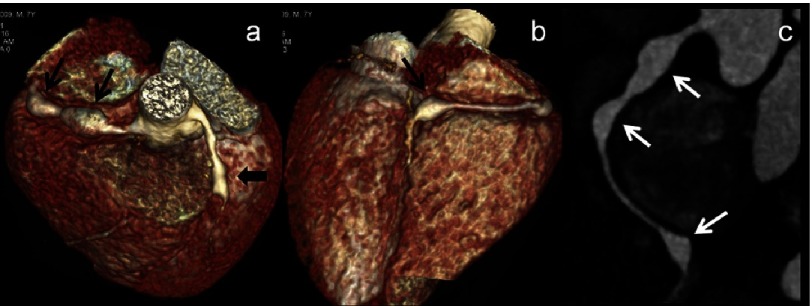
(a–c): CT Coronary angiography (CTCA) images (a & b - volume rendered images-VRT) and (c) curved maximum intensity projection (MIP) images of a 7 year male child in acute phase show two fusiform aneurysms in proximal right coronary artery (RCA) and one in distal RCA at its bifurcation into its posterior descending and postero-lateral branches (thin arrows). Note fusiform aneurysm in mid left anterior descending artery (LAD) as well (a-thick arrow). 2D-transthoracic echocardiography could detect one aneurysm in proximal RCA and proximal LAD and missed two aneurysms in RCA.
